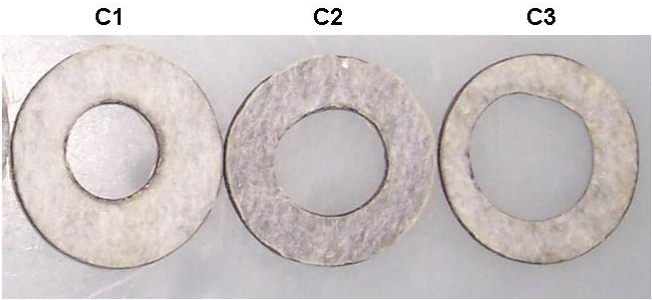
Lead collimators with different diameters (0.5, 1.0, 1.5 cm) and a thickness of 2 mm, used to restrict the X-ray beam onto the tumor lobes.
